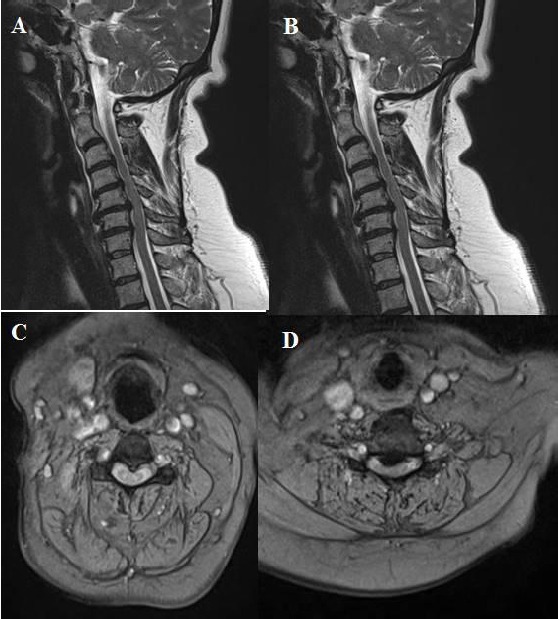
A, B, C, D: T2 weighted sagittal and axial MRI showing Compression the medulla spinalis at two levels that are between cervical 4 and cervical 5, and between cervical 5 and cervical 6. Osteophyte formation and posterior longitudinal ligament ossification lead to this compression on medulla spinalis. Besides, cervical lordosis angle was measured 0 degree on lateral X-ray at the preoperative period*.
